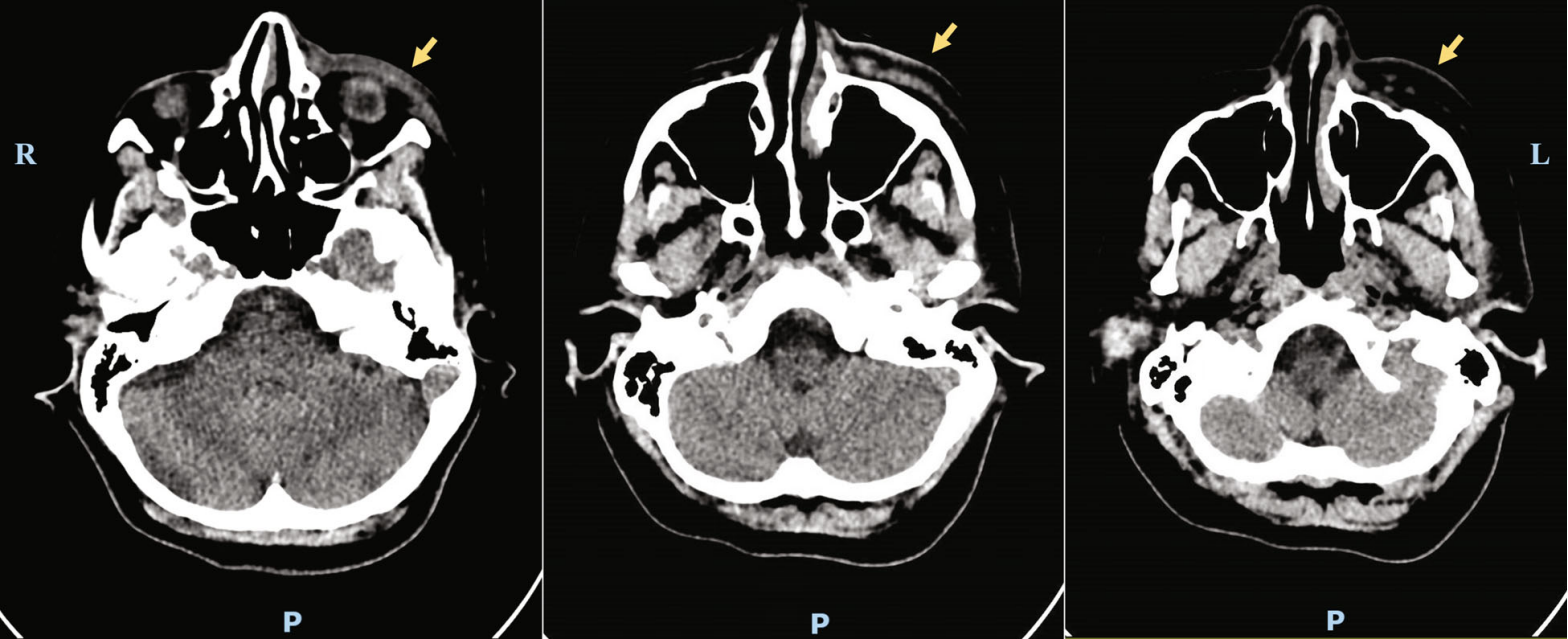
Contrast-enhanced brain angio-CT: normal aspect except for infiltration and thickening of the soft tissues of the left hemiface (yellow arrow).
